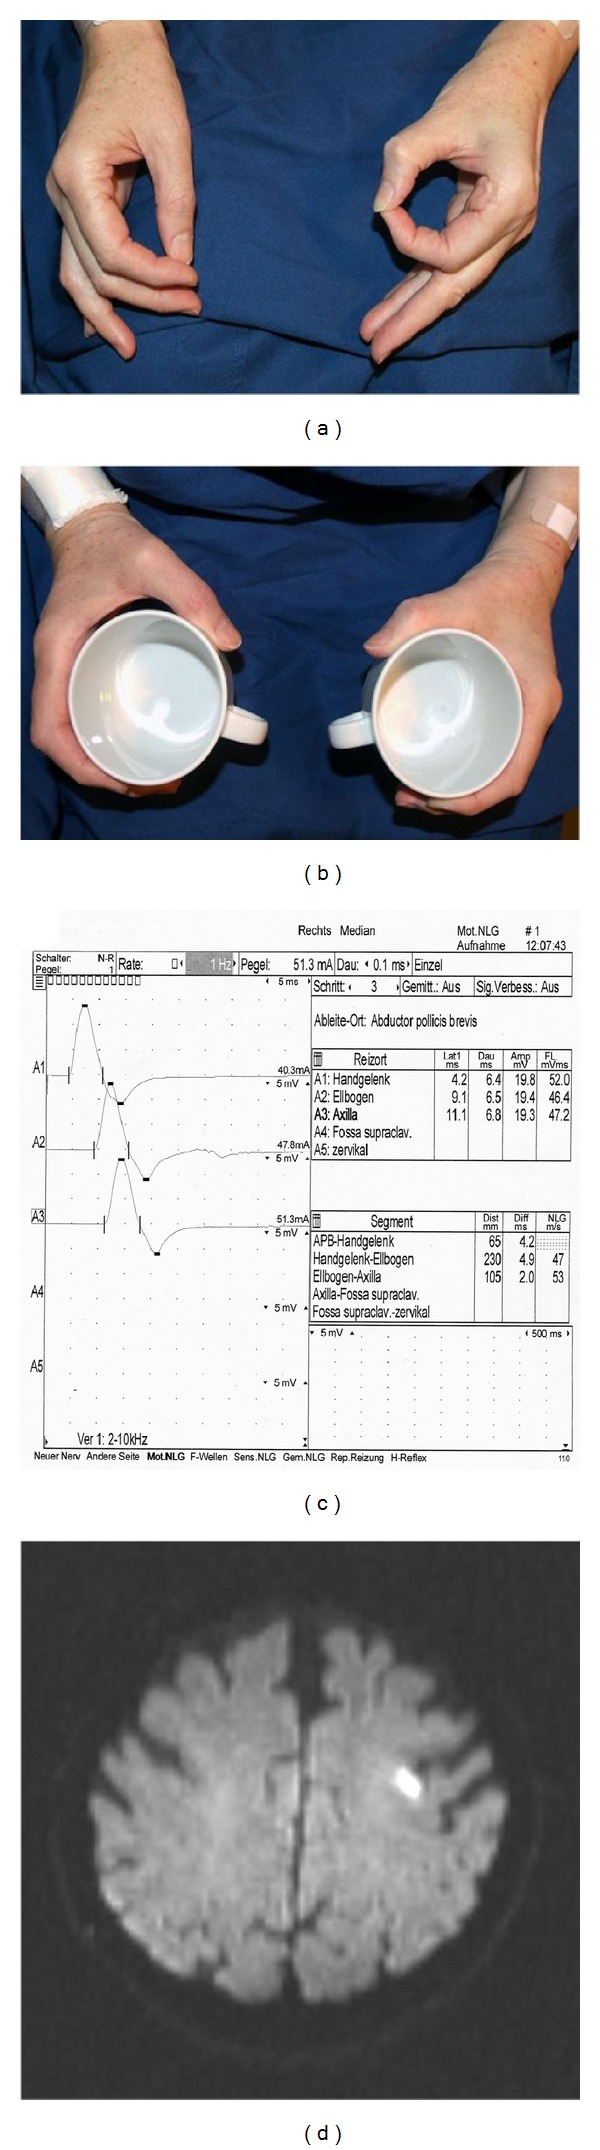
(a) Pathological “circle sign” of the right hand. The patient is unable to correctly oppose the tips of digits I and II due to weakness of flexor pollicis longus muscle and the flexor digitorum profundus muscle of the index finger, (b) Lüthy's bottle sign. See the gap between the cup and the skin web due to weakness of thumb adduction, opposition, and flexion in median nerve lesion, (c) Normal neurography of the right median nerve, (d) Diffusion-weighted magnetic resonance imaging showing an acute infarction in the “hand knob” area of the left precentral gyrus.
